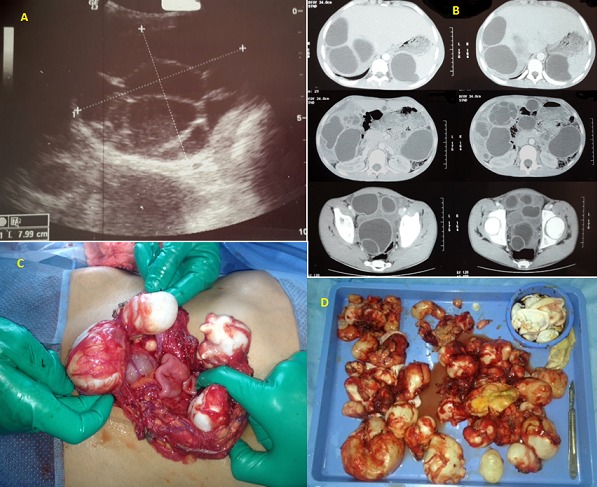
(A) ultrasound image shows a multivesicular hepatic hydatid cyst; (B) CT scan image showing several cysts of the peritoneal cavity; (C) Intraoperative view objectified hydatid cysts of the omentum; (D) all cysts after surgery
